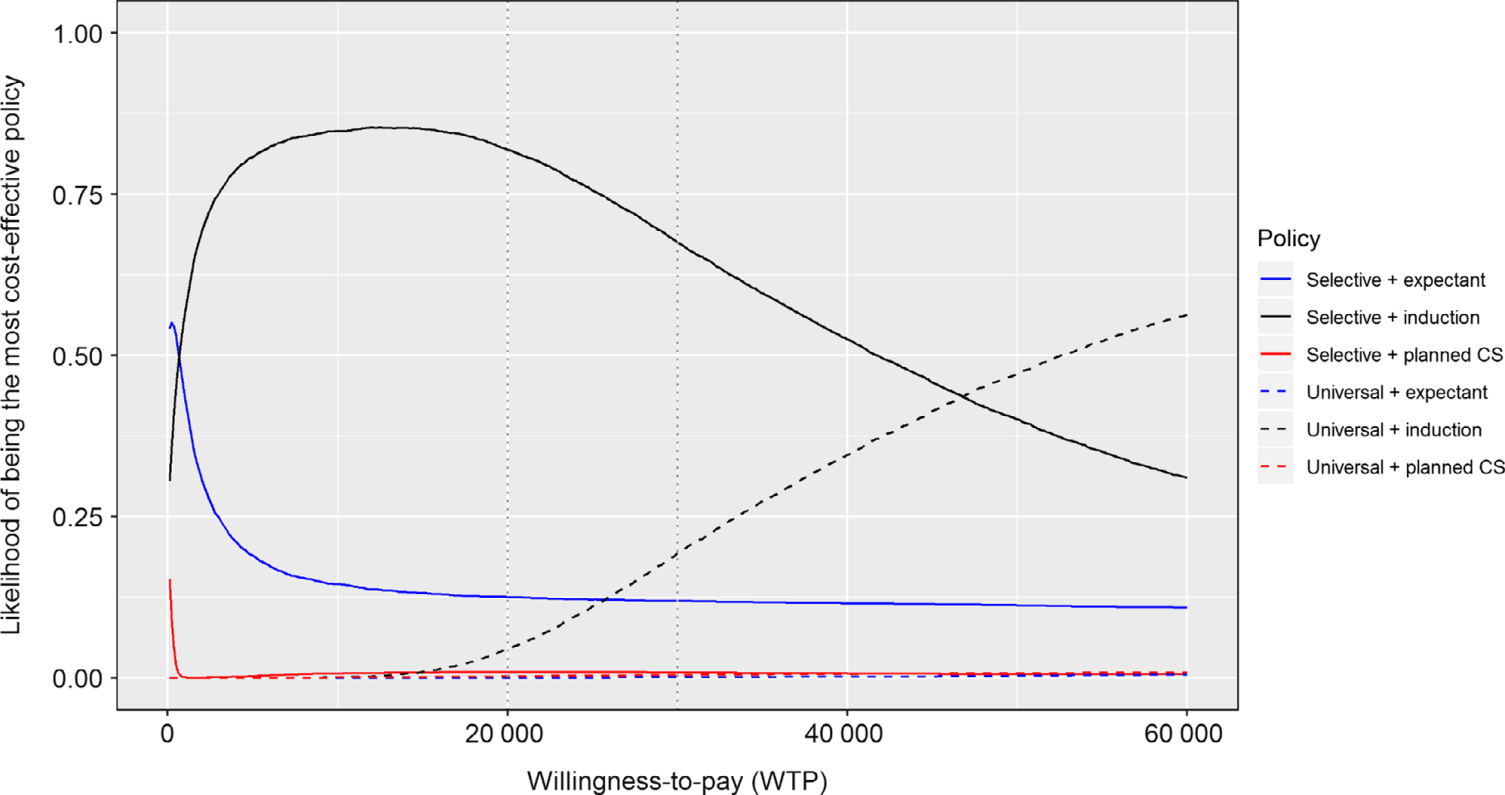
Cost‐effectiveness acceptability curve for policies for detection and management of fetal macrosomia. Cost‐effectiveness acceptability curve showing the chance of each policy of being the most cost‐effective for different levels of WTP. Policies with universal ultrasound are shown as dashed lines and selective ultrasound as solid. Higher values for WTP imply a higher valuation of a QALY. The conventional WTP threshold for cost‐effectiveness is £20,000–£30,000 (marked in figure).^29^
